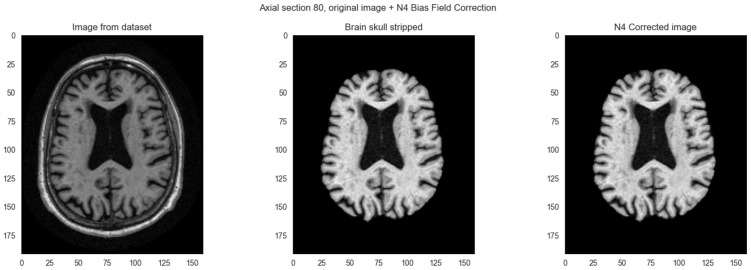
N4 bias field correction on axial section. From left to right: (1) Original MRI image. (2) Skull stripped image showing residual non-uniformity in intensity. (3) Bias field corrected image with normalized intensity across brain regions.
